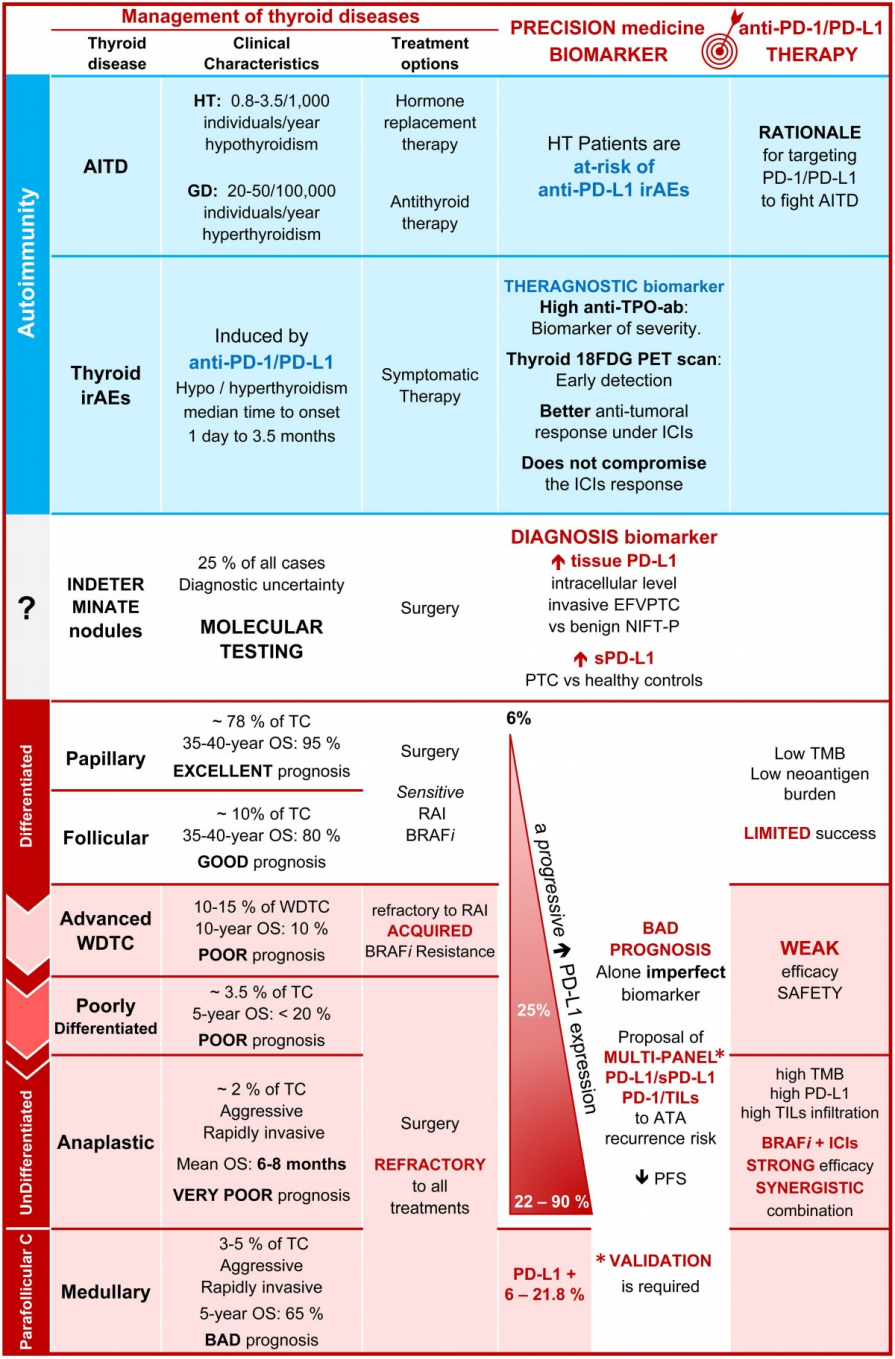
Promise of PD-L1 in the diagnostic, prognostic, and management of thyroid diseases. Comparison of some clinical characteristics, and treatment options, of patients with AITD, irAE, and TC emphasizing the percentage of positive cases for PD-L1, the interest of PD-L1 as a diagnosis and prognostic biomarkers as well as the promises of clinical trials using anti-PD‑1 and anti-PD-L1 drugs (*see for details the tables 1-5*). Abbreviations: 18FDG, 18 fluorodeoxyglucose; AITD, Autoimmune thyroid disease; Anti-TSH-R-ab, Anti-receptor of the thyroid-stimulating hormone antibody; Anti-TPO-ab, Anti-thyroid peroxidase antibody; ATA, American thyroid association; BRAFi, BRAF inhibitor; CLT, Chronic lymphocytic thyroiditis; EFVPTC, Encapsulated follicular variant of papillary thyroid carcinoma; GD, Grave's disease; HT, Hashimoto's thyroiditis; ICIs, Immune checkpoint inhibitors; IFNγ, Interferon-gamma; irAEs, Immune-related adverse events; NIFTP, Non-invasive follicular thyroid neoplasm with papillary-like nuclear features; OS, Overall survival; Parafollicular C, Parafollicular carcinoma; PD-1, Programmed-cell death receptor 1; PD-L1, Programmed-cell death ligand 1; PTC, Papillary thyroid carcinoma; RAI, Radioiodine therapy; SNP, Single nucleotide polymorphism; TC, Thyroid carcinoma; TILs, Tumor-infiltrating lymphocytes; PD-1, Programmed-cell death receptor 1; PD-L1, Programmed-cell death ligand 1; PFS, Progression-free survival; SNP, Single-nucleotide polymorphism; TMB, Tumor mutational burden; WDTC, Well-differentiated thyroid carcinoma.
